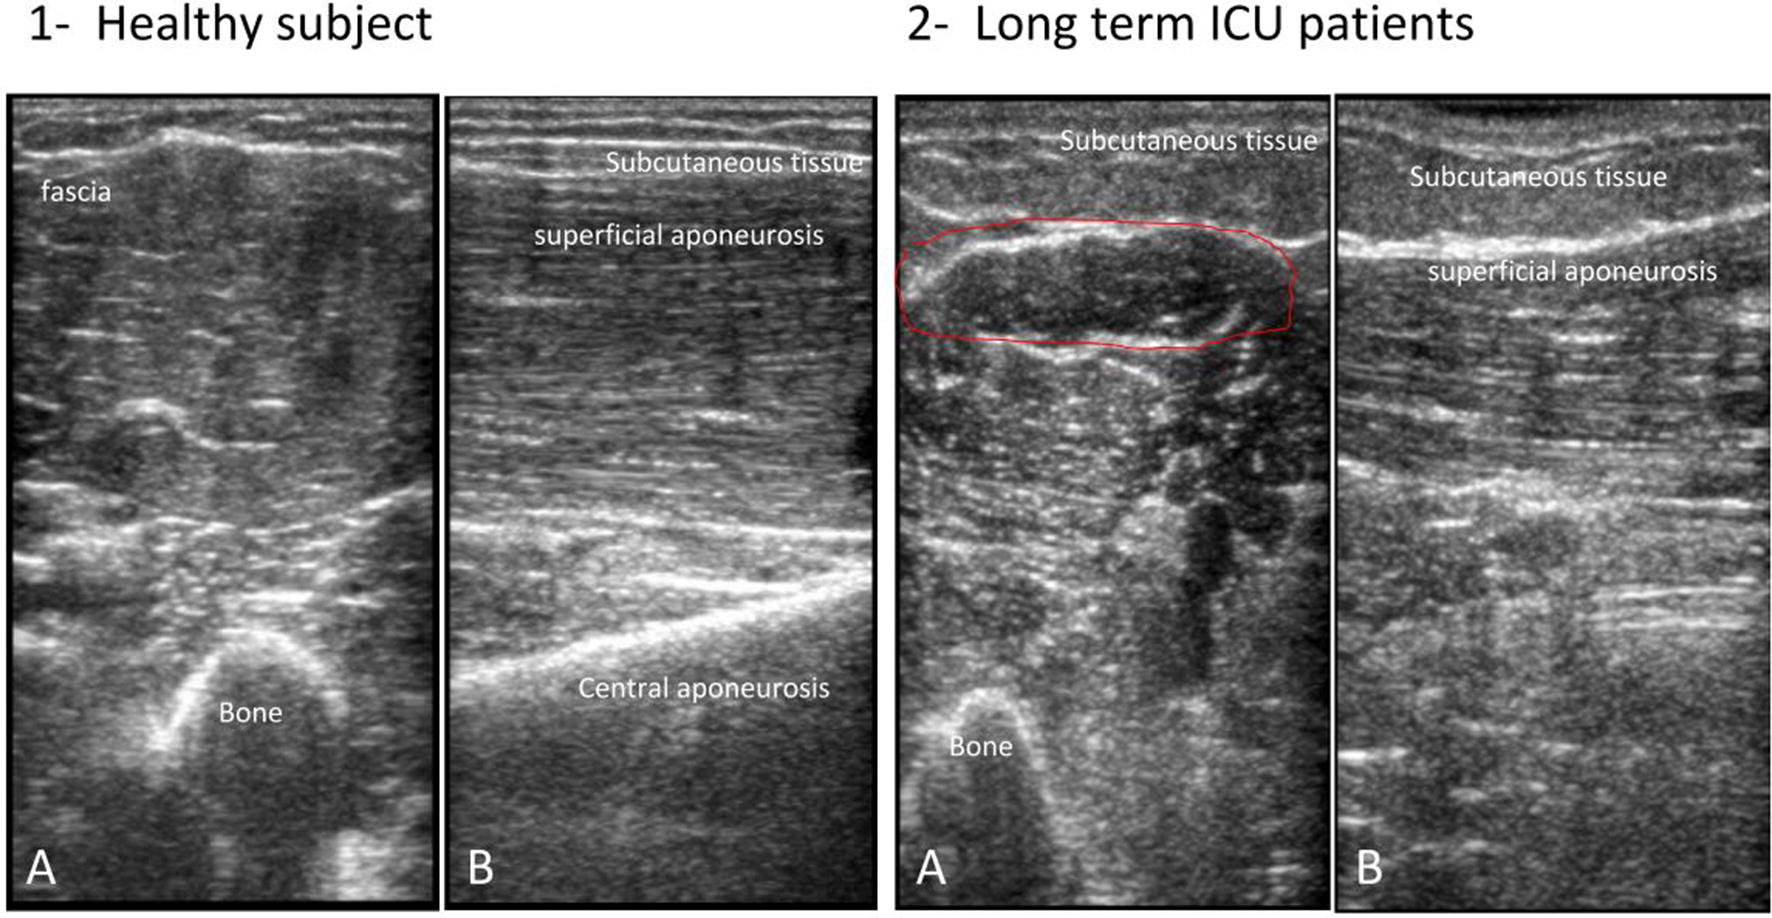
Ultrasound distinctive appearance of muscle tissue. The figure shows a transverse (a) and longitudinal (b) ultrasound scan of elbow flexor (bicep brachialis) in healthy (1) and long-term ICU (2) subjects. In the axial image, muscle consists of primarily hyper-echogenic areas scattered with small bright curved echoes of superficially random orientations. In the sagittal plane, these bright echoes are seen to be the fibrous tissue that surrounds muscle fibers and fascicles and which organize into recognizable striations. In bipennate or multi-pennate muscles, a central aponeurosis can be identified as an area of thickened fibrous tissue that when followed distally becomes the tendon. Bone is highly echogenic with a deep shadow beneath the bright hard edge. Subcutaneous fat is typically of similar echogenicity to muscle and is interposed with brighter, poorly organized strips of connective tissue. Near the myotendinous junction, the myofascial fibrils merge, resulting in increased echogenicity and higher anisotropy. In the healthy tissue, the hyper-echogenic muscle is interspersed with bright fibro adipose tissue and the bone reflection is bright and sharply defined; in the long-term ICU patient, the muscle tissue appears as non-homogenous and reduced in its mass
